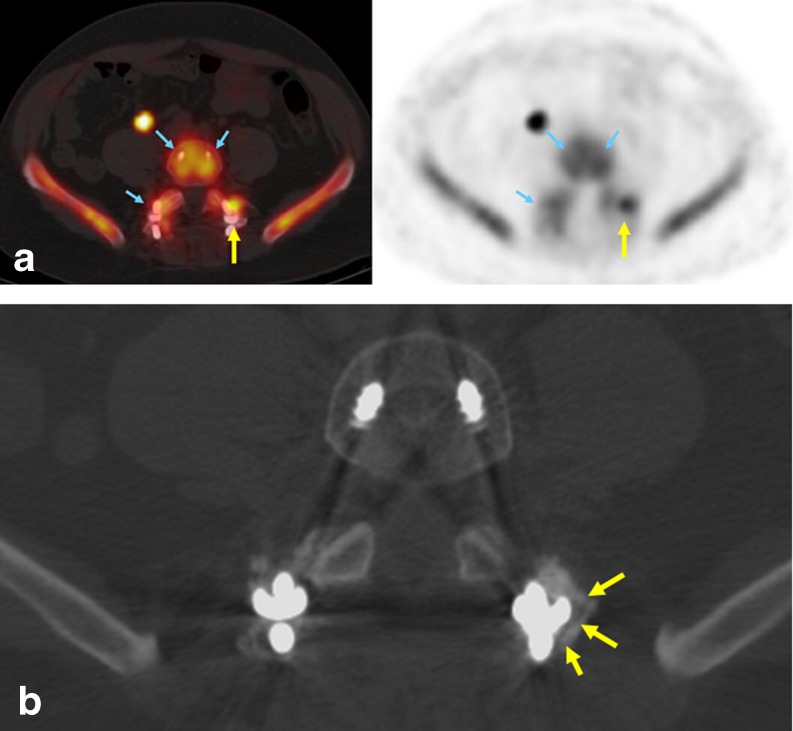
PET/CT images in patient 16 a Intense and asymmetric ^18^F-NaF activity is apparent in the left L5 screw (*yellow arrow*, SUV 7.4). Relatively normal tracer activity is apparent in the other hardware (*blue arrows*, SUV 5.2–5.5). Left L5 screw loosening was confirmed on subsequent surgical exploration. b Zoomed CT image of the left L5 screw demonstrates possible radiolucency around the screw (*yellow arrows*)
